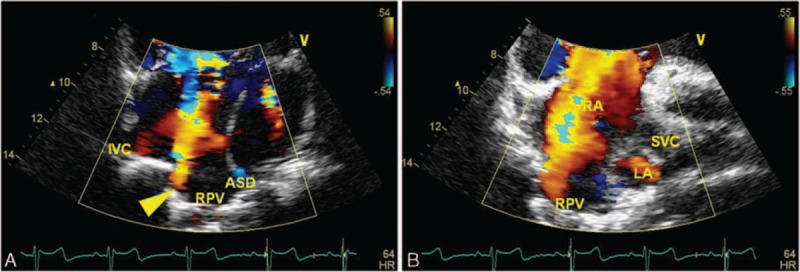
Color Doppler of the right pulmonary vein draining into the right atrium (RA) of a 13-year-old boy. (A) Apical 4-chamber view shows the right pulmonary vein (RPV) and inferior vena cava draining into the RA and the atrial septal defect located at the top of the atrial septum. (B) The apical rotation view shows the superior vena cava and RPV draining into the RA.
